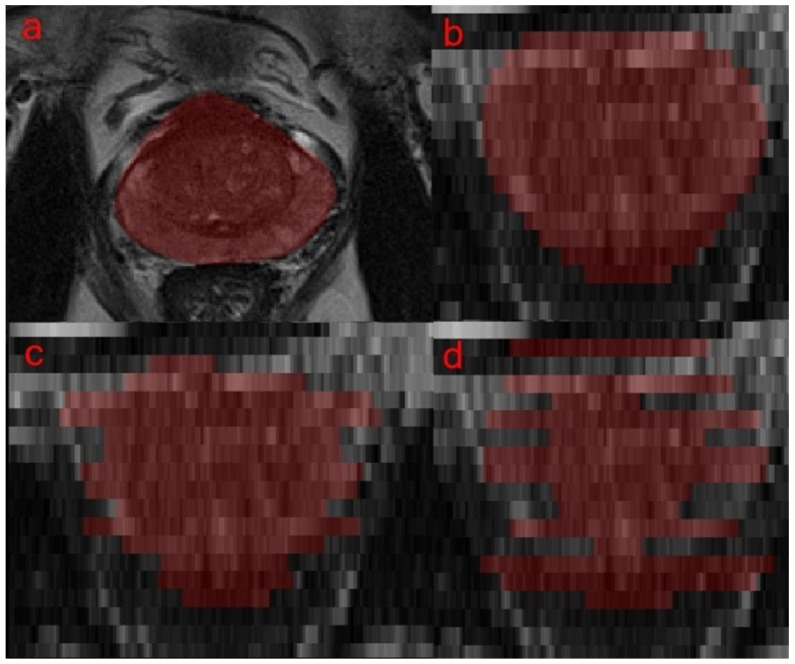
Image panels (a–d) denote prostate volume segmentations; (a) unmodified RS segmentation on a T2-weighted axial image; (b) unmodified RS segmentation on a T2-weighted coronal image; (c) training set segmentation with a potential 5 mm modification in either the inner or outer radial direction on each axial slice as seen on a T2-weighted coronal image; and (d) training set segmentation with a potential 10 mm modification in either the inner or outer radial direction on each axial slice as seen on a T2-weighted coronal image.
